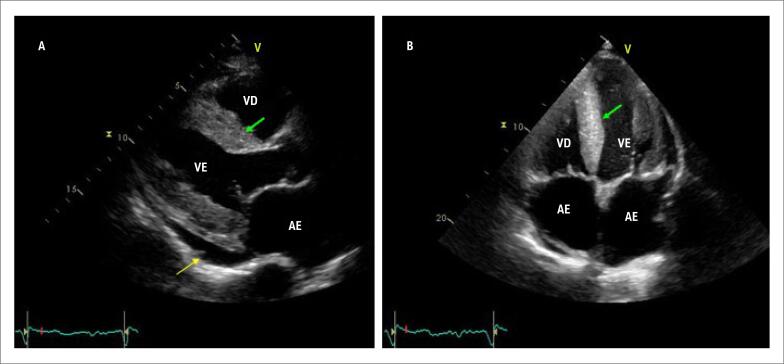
Apresentação ecocardiográfica clássica de amiloidose cardíaca (AC). Em A, a projeção paraesternal longitudinal apresenta o ventrículo esquerdo (VE) de tamanho normal, mas com espessura de paredes aumentada e aspecto granulado em septo interventricular (seta verde). Há, ainda, sinais de aumento do átrio esquerdo (AE) e leve derrame pericárdico (seta amarela). Em B, a projeção apical 4c evidencia os átrios grandes, ventrículos de dimensões normais e aumento de espessura de paredes, também com aspecto granulado do septo interventricular (seta verde). As valvas mitral e tricúspide são levemente espessadas. VE: ventrículo esquerdo; VD: ventrículo direito; AD: átrio direito. (Imagens de arquivo pessoal dos autores).
